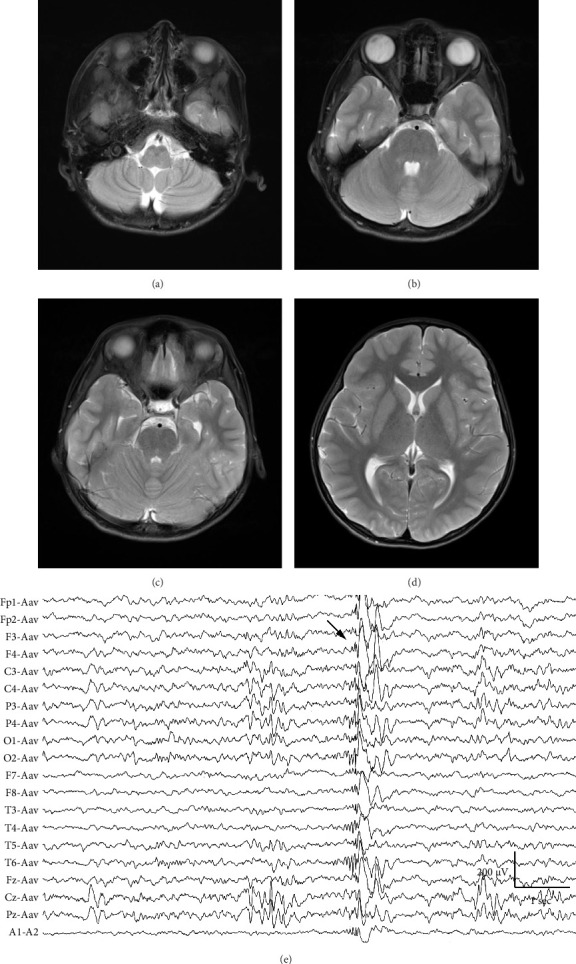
Diagnostic work-up of syncope with brain MRI (a–d) and electroencephalography (e). Axial T2-weighted images at the level of the medulla (a), cerebellum (b), pons (c), and basal ganglia (d) showing no white or gray matter abnormalities. (e) Example of interictal discharges on the average reference montage of a drowsy EEG. Right frontal dominant irregular, diffuse spike-waves, and polyspike-waves are shown (arrow).
